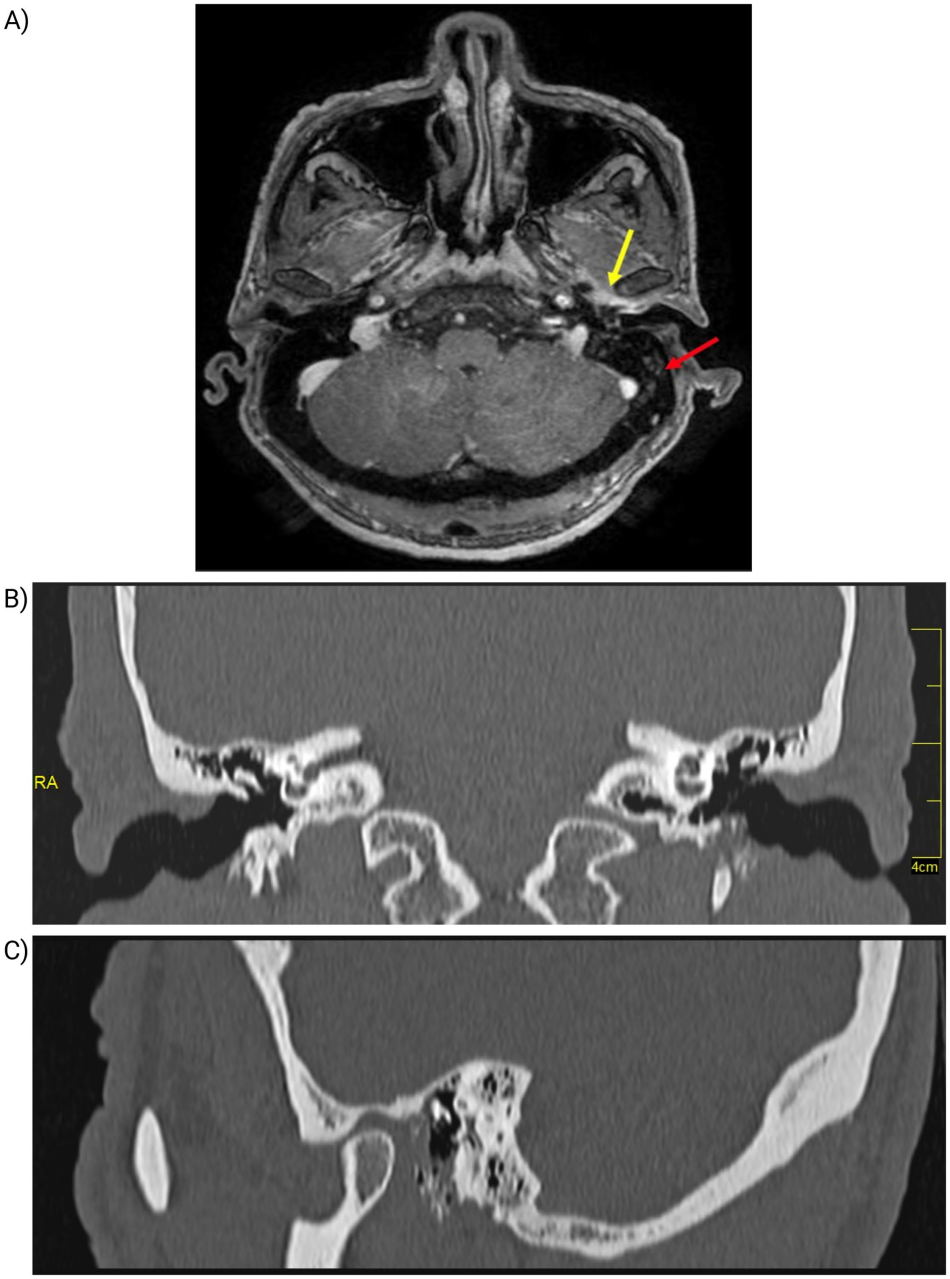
Imaging findings of the left ear. (A) Magnetic resonance imaging (MRI) of the left ear. Demonstrating opacification of the mastoid air cells (red arrow) and altered signal intensity at the inferior margin of the external auditory canal (yellow arrow). (B,C) Computed Tomography of the Left Ear. (B) Coronal CT scan shows bone erosion in the anteroinferior and inferior walls of the external auditory canal (red arrow). (C) CT scan shows soft tissue density with bone involvement (yellow arrow).
